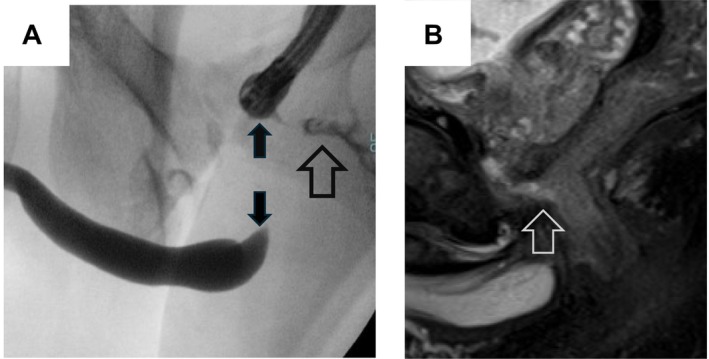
(A) Combined urethrogram shows urethral gap and urethrorectal fistula. (B) T2‐weighed magnetic resonance imaging showing urethrorectal fistula. Open arrow: Urethrorectal fistula; Closed arrow: Urethral gap.
